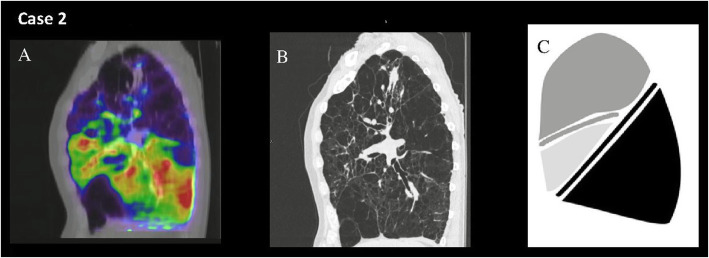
Highlighted are sagittal fused 68Ga-VQ/PET-CT (a), CT densitometry (b), and StratX® report (c) images from a participant (case 2) demonstrating discordance in modalities in the lower lobes, with high perfusion to lower lobe despite high destruction scores based on CT densitometry. Panel A depicts the right lung with perfusion in color (RUL 42%, RML 12%, RLL 14%), with b showing the CT density. Panel C It indicates right lower lobe having the highest destruction score (70%) despite functional imaging suggesting it had an adequate level of perfusion with one of the higher lobar percentages of normal ventilation/perfusion (30%)
